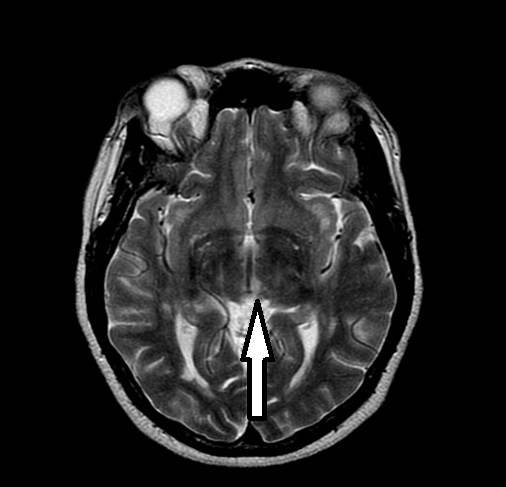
T2 fluid attenuated inversion recovery (FLAIR) image of the left medial thalamic infarct.
